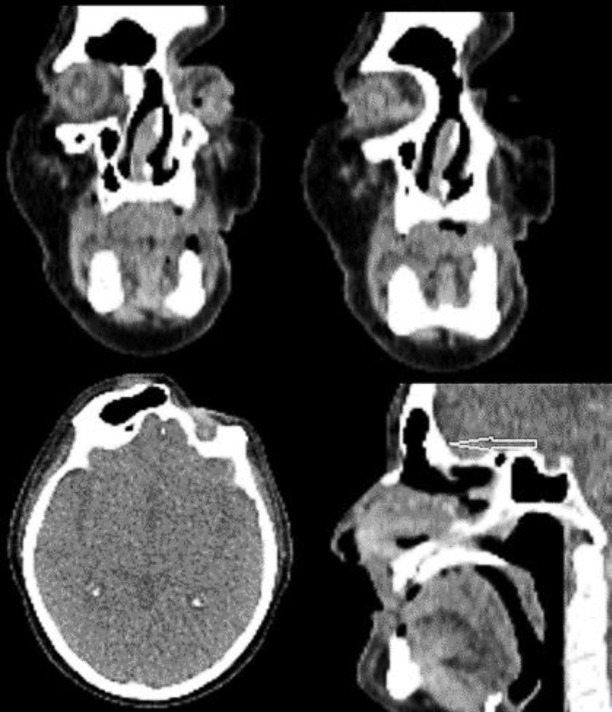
Paranasal sinus two-month CT scan after operation (Modified lothrop procedure condition): free frontoethmoidal area with complete right frontal drainage. Remnants of frontoethmoidal bone hyperostosis (white arrow) on anterior cranial fossa wall
